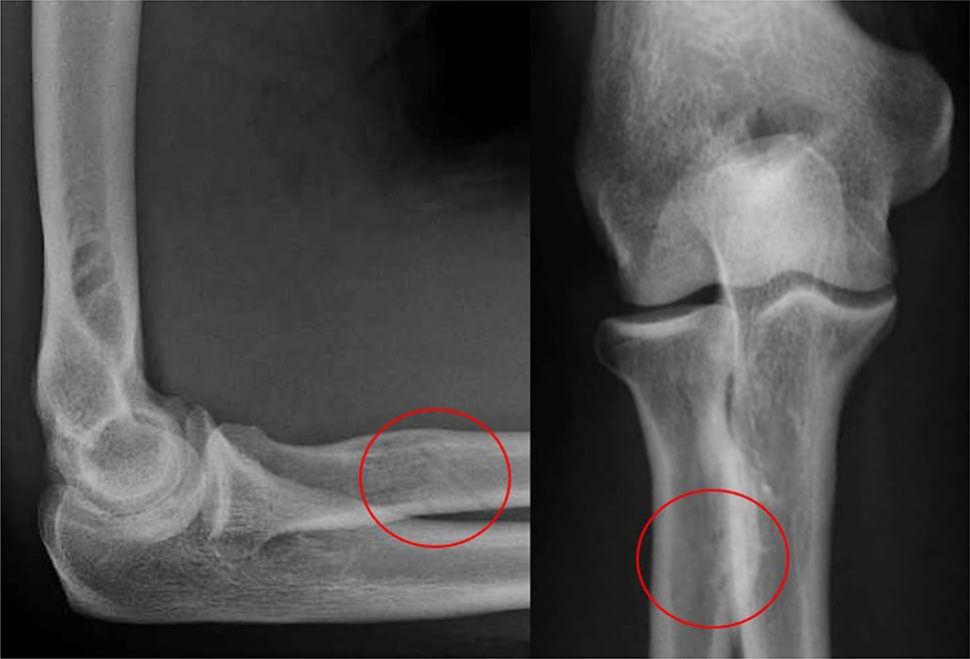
The drill holes in the radial tuberosity can be seen on the postoperative X-rays
